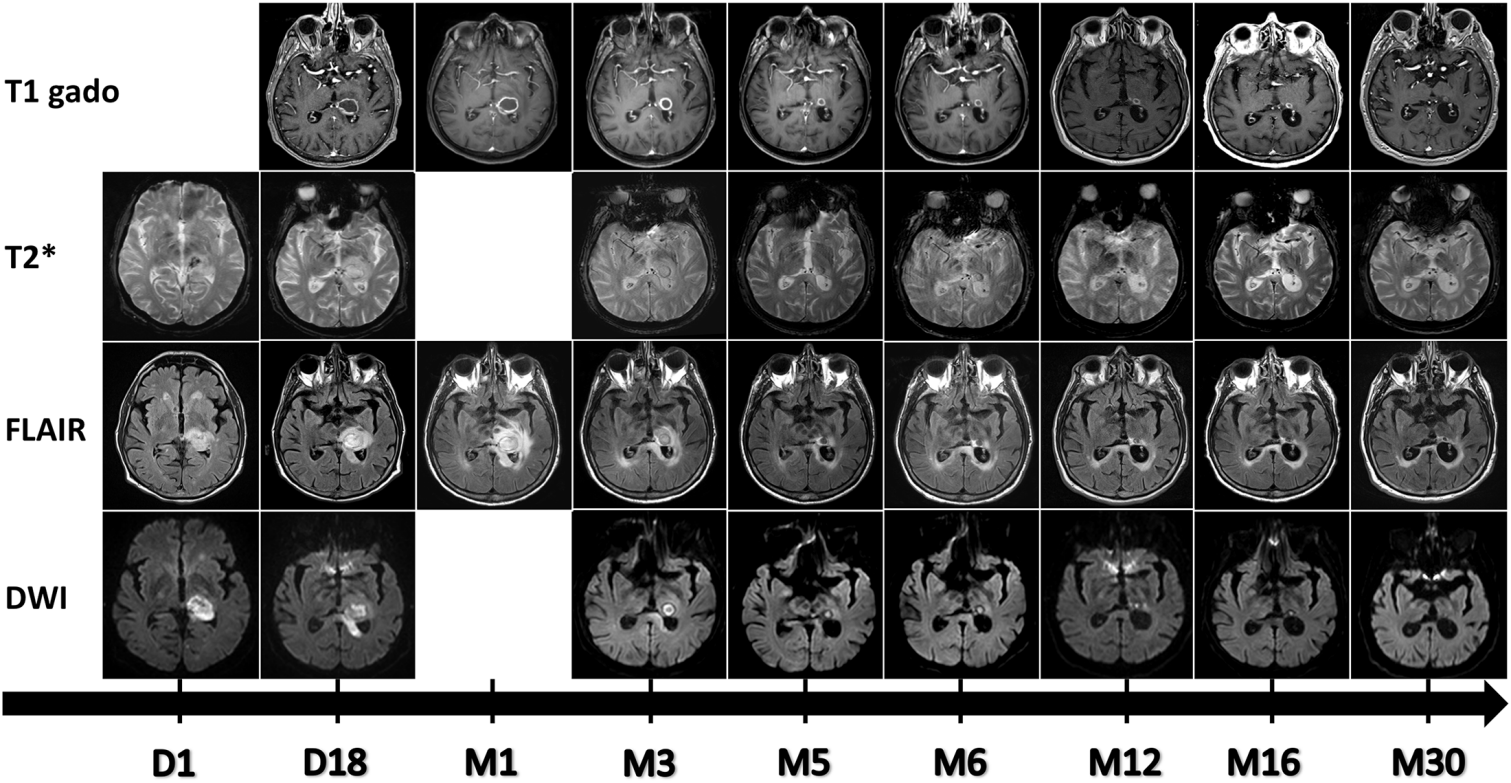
Evolution of a left thalamic abscess (patient #10) from day 1 to month 30. T1 after gadolinium injection, T2*, FLAIR and Diffusion-weighted images show annular peripheral enhancement with central necrosis and a progressive decrease in size starting at month 3.
